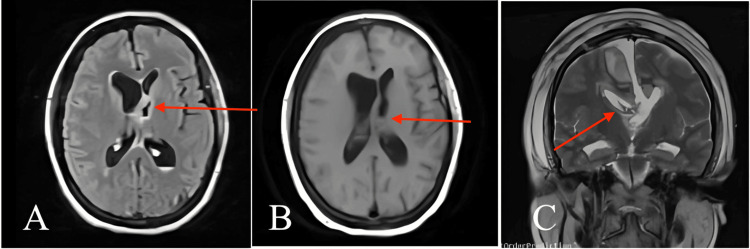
Postoperative MRI of the brain (A) axial T2 FLAIR showed gross total resection of the cyst (red arrow); (B) axial T1 showed no evidence of residual tumor or cyst (red arrow); (C) coronal T2 image shows the directory of the surgical approach and confirms the gross total resection and delineate the cyst cavity (red arrow). FLAIR - fluid-attenuated inversion recovery
